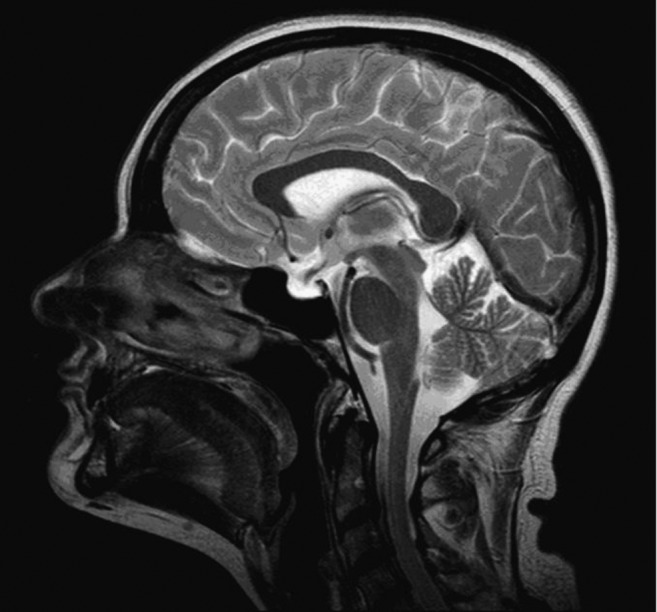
Sagittal T2-weighted MRI head – appearances consistent with empty sella turcica.
